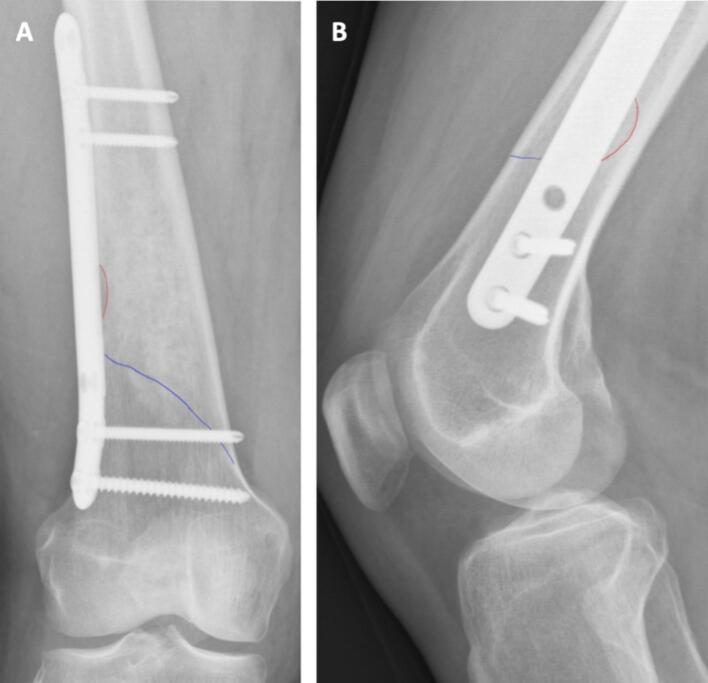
AP (A) and lateral X-ray images (B) of a fissure (blue line) after curettage with prophylactic plate fixation. The fissure occurred despite the presence of the plate fixation. The red line indicates the oval shaped cortical window. (For interpretation of the references to colour in this figure legend, the reader is referred to the web version of this article.). (For interpretation of the references to colour in this figure legend, the reader is referred to the web version of this article.)
